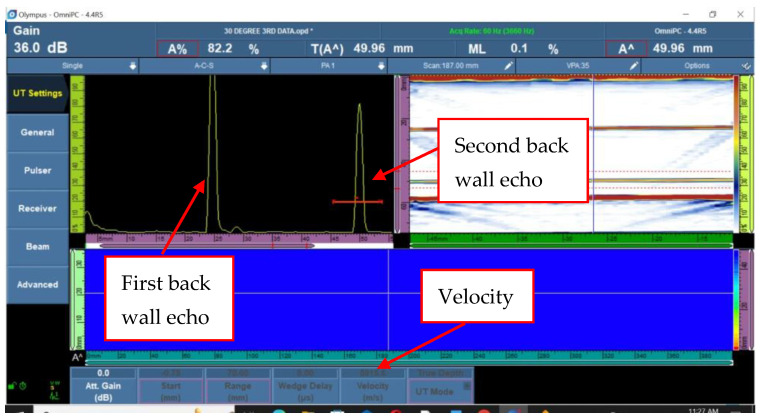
Ultrasonic velocity and decibels by experiment.
